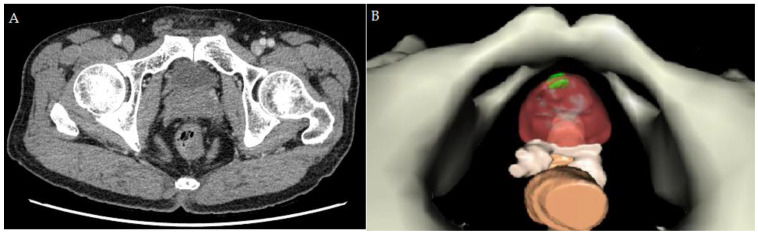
(A) The 1.25-mm thin slice for computed tomography used for reconstruction. (B) The reconstructed personal 3D image using for RAPP. The green area indicates the location of PCa.
